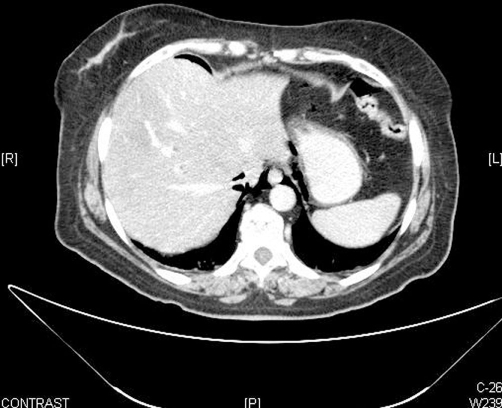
Abdominal CT scan revealed extraperitoneal air from the sigmoid perforation diffusing superiorly through paravertebral retroperitoneal tissue and via the diaphragmatic hiatus into the mediastinum.
